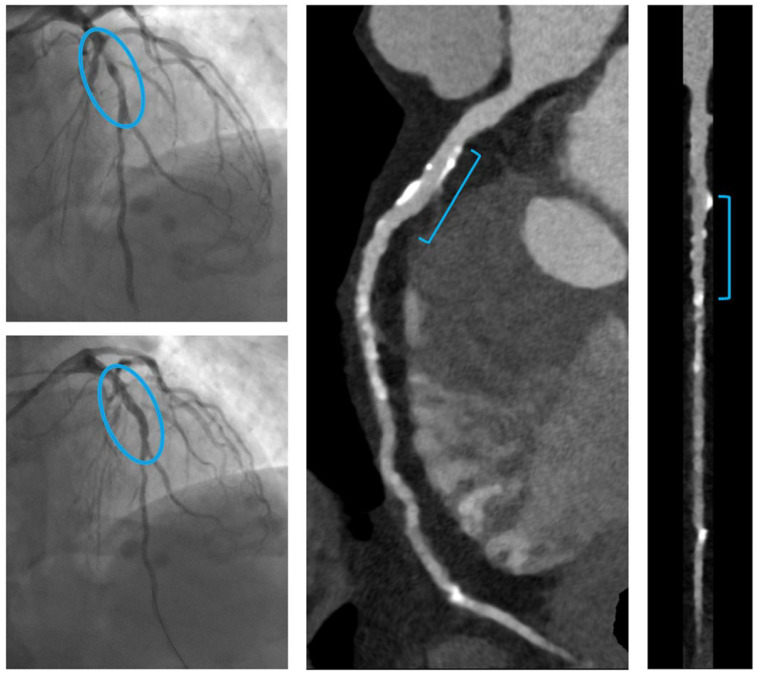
Example of no completely reabsorbed RMS. On the left, from top to bottom, angiographic images show a focal plaque in the middle tract of the LAD, successfully treated by implantation of one RMS (Magmaris^®^ 3x25 mm). Scaffold struts are visible in the CT reconstruction: they appear as bright linear fragments along the treated vessel tract. The colored bar in the FFR-CT image provides a map of FFR-CT values, with blue and green indicating non-significant stenosis.
